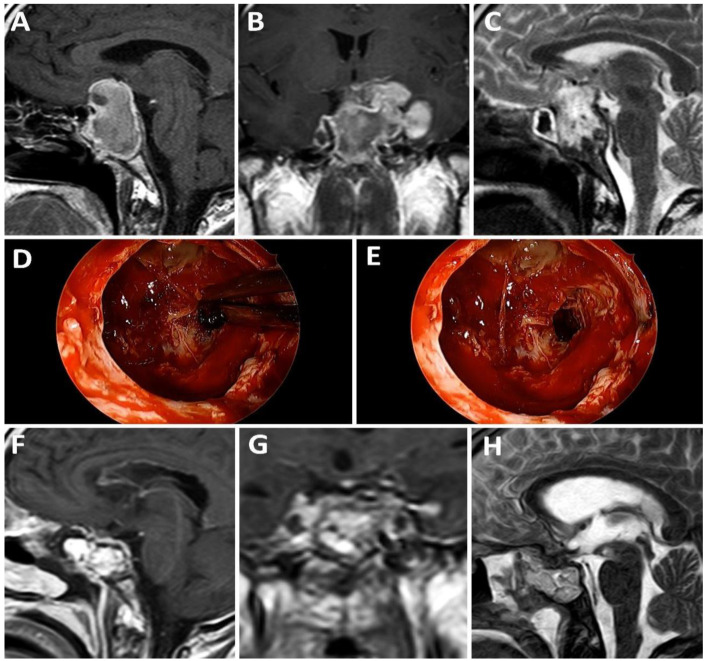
A 23-year-old patient with pituitary apoplexy in the context of a giant prolactinoma. (A–C): Preoperative MRI; (D,E): intraoperative findings; (F–H): postoperative MRI.
